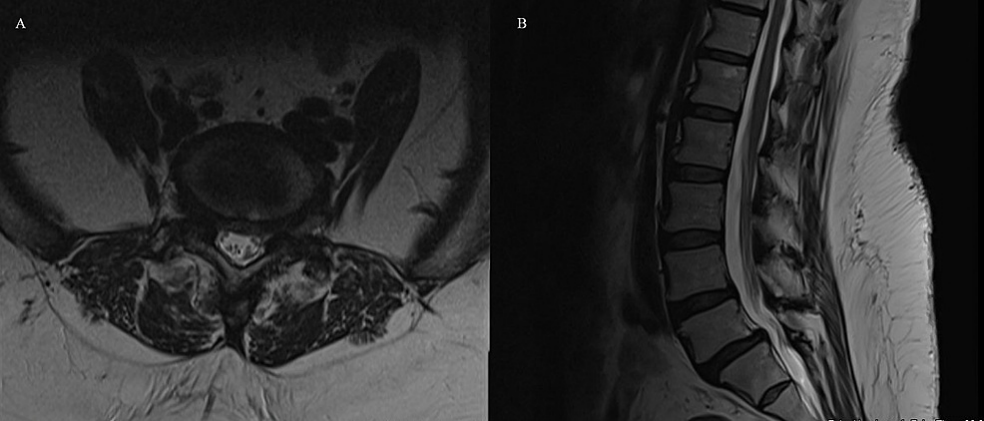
Axial (A) and sagittal (B) T2 weighted MRI of the lumbar spine demonstrating L4/5 and L5/S1 grade 1 spondylolisthesis with foraminal stenosis and minimal central stenosis
